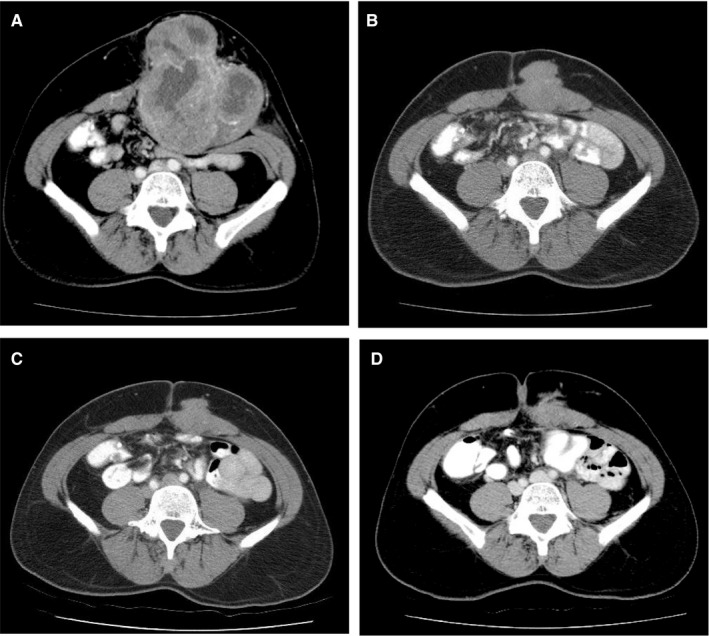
Sustained response of DF to MTX/VNL on MRI imaging. Response of DF on MRI throughout treatment with MTX and VNL, showing continued response despite treatment stopping after 25 cycles. A. Pretreatment, B. After 12 cycles, C. Posttreatment, 1 month after treatment end, D. Posttreatment, 2 months after treatment end
